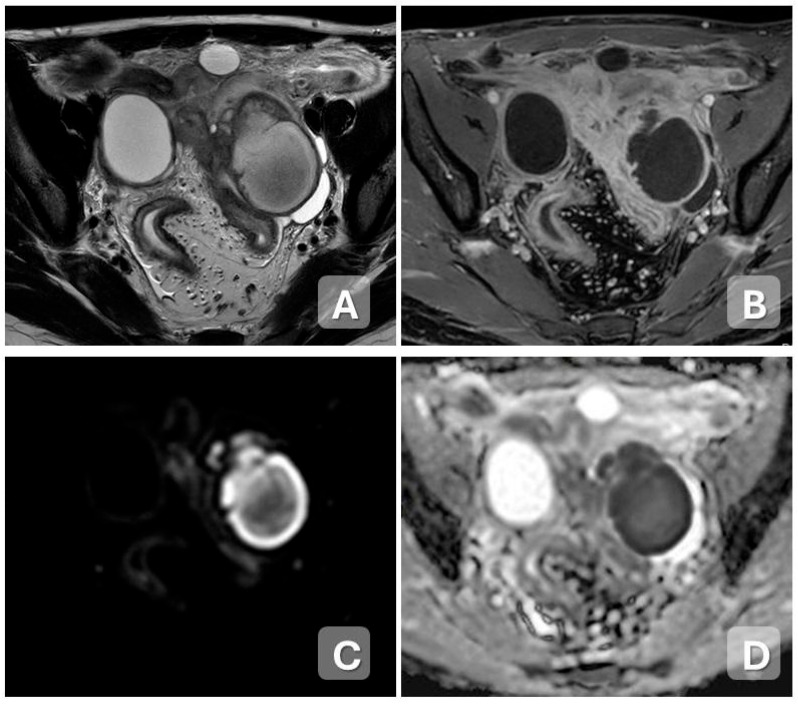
Tubo-ovarian abscess in a 30-year-old woman with worsening pelvic pain. An increase in volume of the left adnexa and left Fallopian tube, due to the presence of a formation with fluid-corpuscular content (A), showing restriction in DWI (C) and ADC (D), and homogeneous post-contrast graphic enhancement of the walls of the formation (B).
